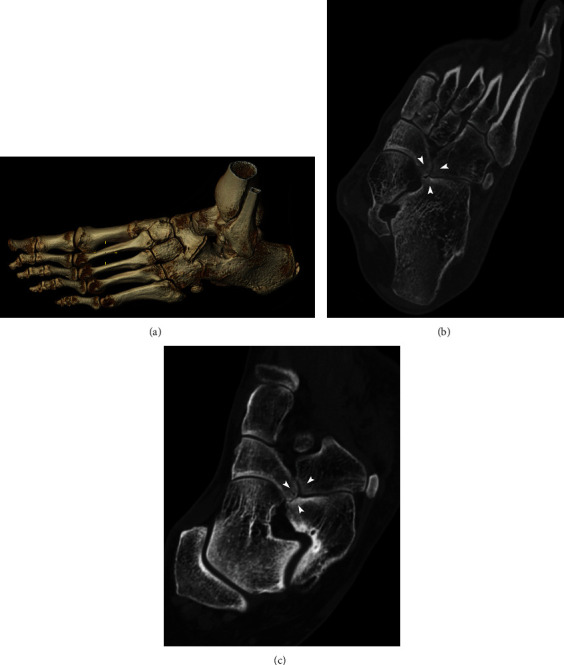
Dorsolateral volume rendered CT image of the left foot (a) shows hypertrophic arthritis changes between calcaneus and navicular due to nonosseous calcaneonavicular coalition. Oblique multiplanar reformatted CT images of the left foot (b-c) show a nonosseous calcaneonavicular coalition associated with a cuboidonavicular coalition (arrowheads), with degenerative changes between these tarsal bones.
